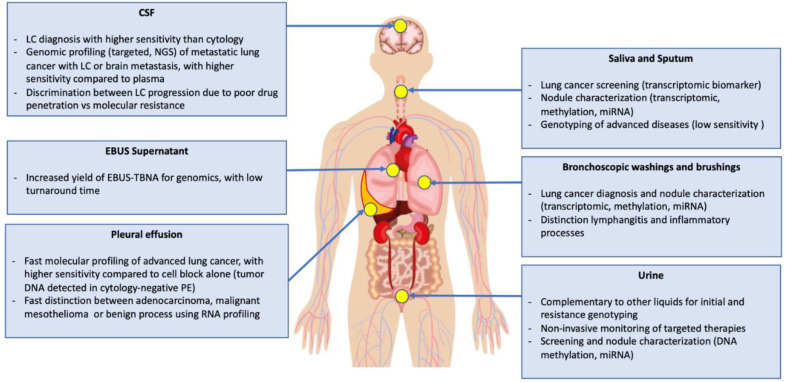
Easily available body fluids and their potential applications. BW: bronchial washing, PE: pleural effusion, CSF: cerebrospinal fluid, LC: leptomeningeal carcinomatosis, EBUS-TBNA: endobronchial ultrasonography transbronchial needle aspiration, NGS: next generation sequencing.
